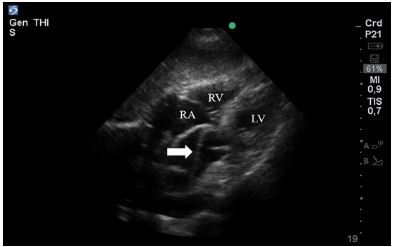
Transthoracic echocardiography subxyphoid view of a patient in post-cardiac arrest with a dissection flap (white arrow)visualized in the ascending aorta. *LV*, left ventricle; *RV*, right ventricle; *RA*, right atrium.
